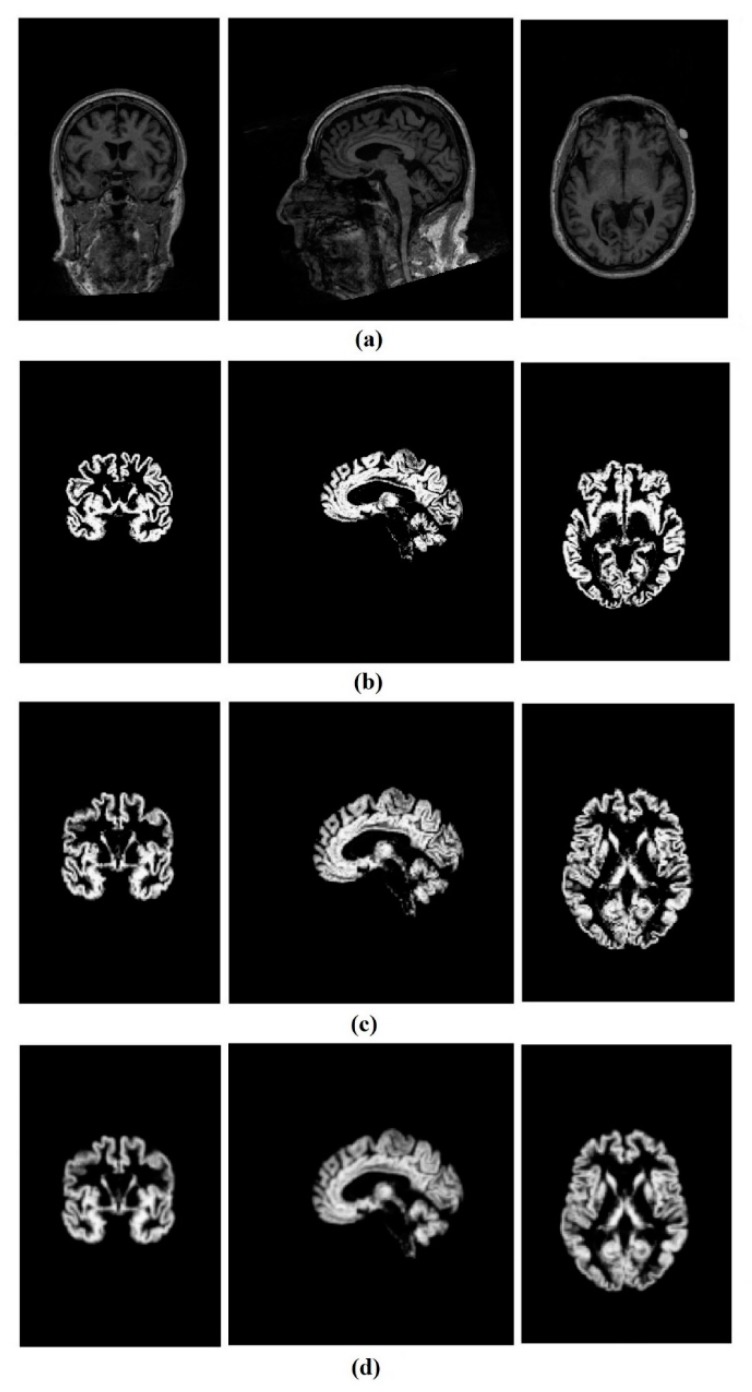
A control healthy subject’s MRI, (a) from left to right sagittal, coronal and axial view, (b). gray matter, (c). gray matter after normalization, (d). gray matter after smoothing.
